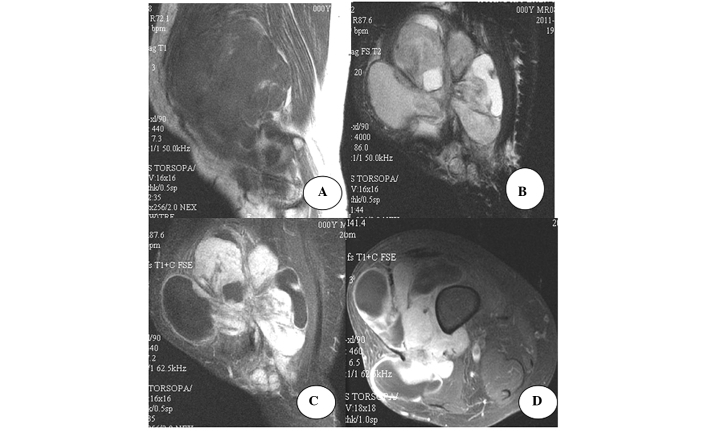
A 51-year-old female with synovial sarcoma. (A–D) Magnetic resonance imaging revealing a well-defined, lobulated soft-tissue mass near the right knee. A slightly high-intensity signal relative to muscle is present on T1-weighted imaging (T1WI) and T2WI. High- and low-intensity signals are also present. Contrast-enhanced scan reveals heterogeneous enhancement, with no clear enhancement observed in areas of necrosis or internal septation.
